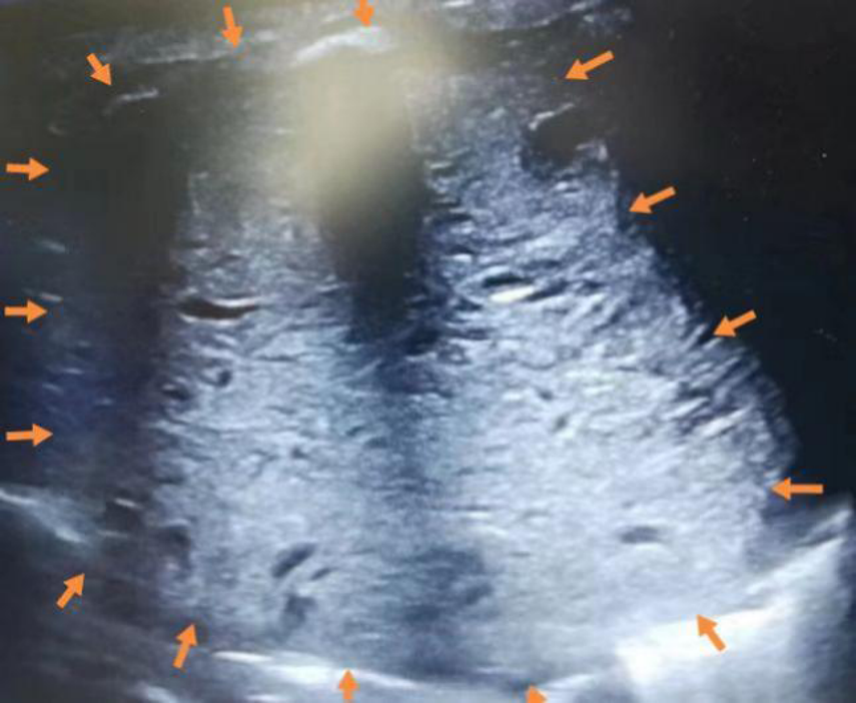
Postnatal ultrasound showing a well-circumscribed, isoechoic mass (arrows) (5.7 × 5.5 × 6.1 cm^3^) with anechoic cystic spaces.
